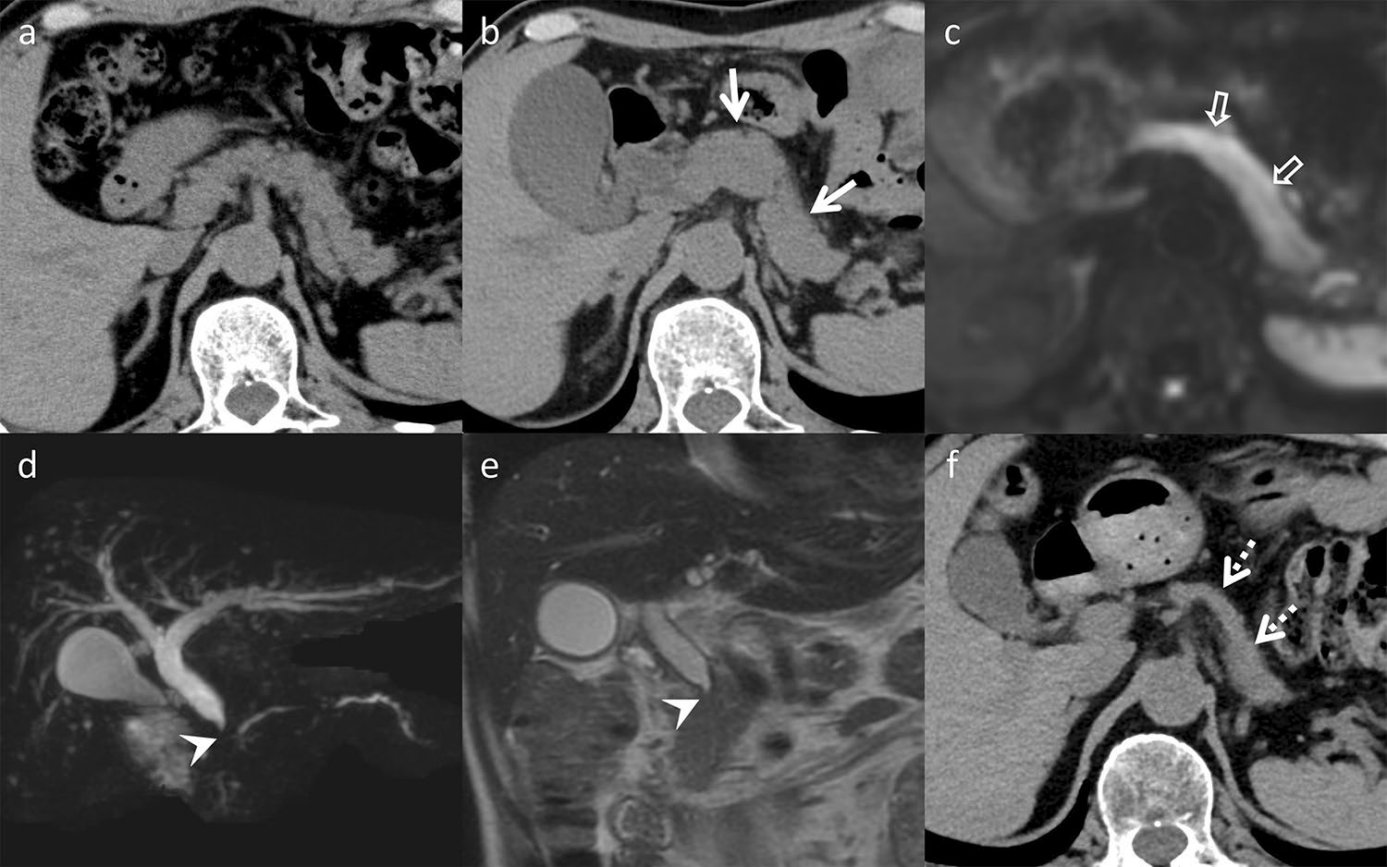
Immune-related adverse events in an asymptomatic 65-year-old man, who was undergoing chemotherapy (CTX, nivolumab) for bone metastases from renal cell carcinoma after a nephrectomy. a Unenhanced computed tomography (CT) reveals no pancreatic abnormalities one year following the initial CTX. Two years after the initial CTX, pancreatic enlargement, loss of lobulation (arrows) on b unenhanced CT, and diffuse hyper-intensity on c diffusion-weighted imaging (open arrows) are detected. Additionally, obstruction and wall thickening of the common bile duct are noted on d magnetic resonance cholangiopancreatography and e T2-weighted imaging (arrowheads). The pancreas is atrophic after steroid therapy on f unenhanced CT (dotted arrows). On imaging, immunoglobulin G4-related disease is a differential; however, it is difficult to differentiate immune-related adverse events from immunoglobulin G4-related disease on imaging alone
